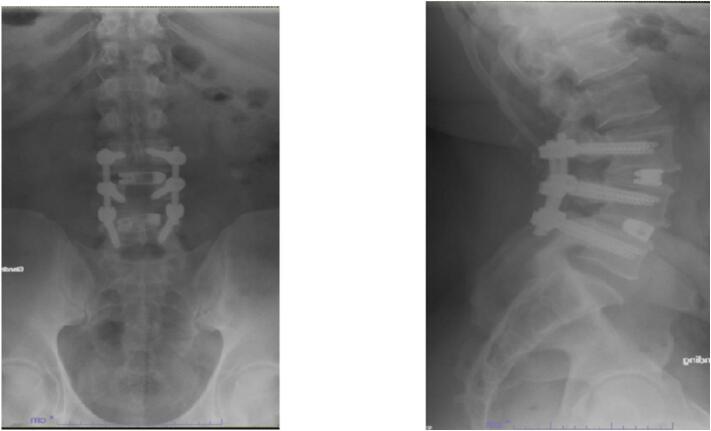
2/1/22- Postoperative Anterior/Posterior and Lateral Radiographs demonstrating intact L3–5 posterior decompression and instrumented fusion with L3–4, L4–5 transforaminal interbody fusion.
